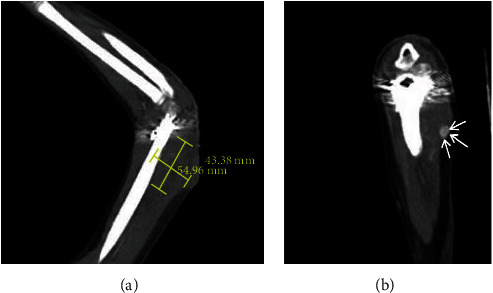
(a) Coronal cut of CT angiography demonstrating maximum diameter of sac of pseudoaneurysm originating from left profunda brachii artery. (b) Extravasation of contrast in pseudoaneurysm of profunda brachii artery in CT angiography.
